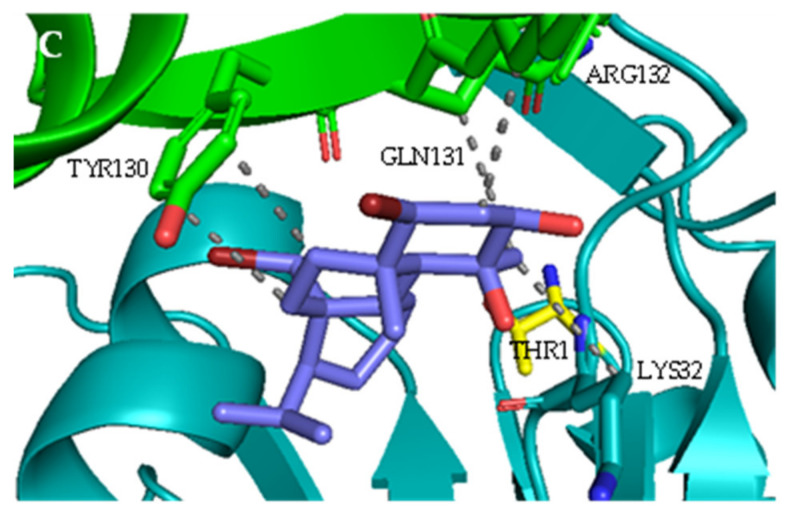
Docking poses of the *Sphaerococcus coronopifolius* bromoditerpenes in the proteasome chymotrypsin-like (CT-L) active site (β5 subunit: cyan; β6 subunit: green; catalytic THR1: yellow). (A) Bromosphaerol, (B) 12*S*-hydroxy-bromosphaerol, (C) 12*R*-hydroxy-bromosphaerol.
